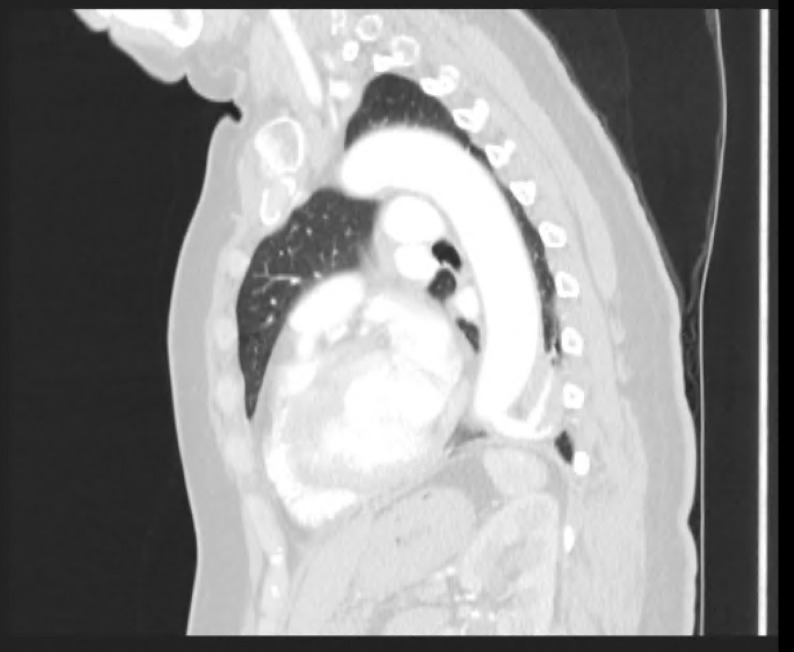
CT Chest with contrast sagittal view showing feeding vessel from descending thoracic aorta
